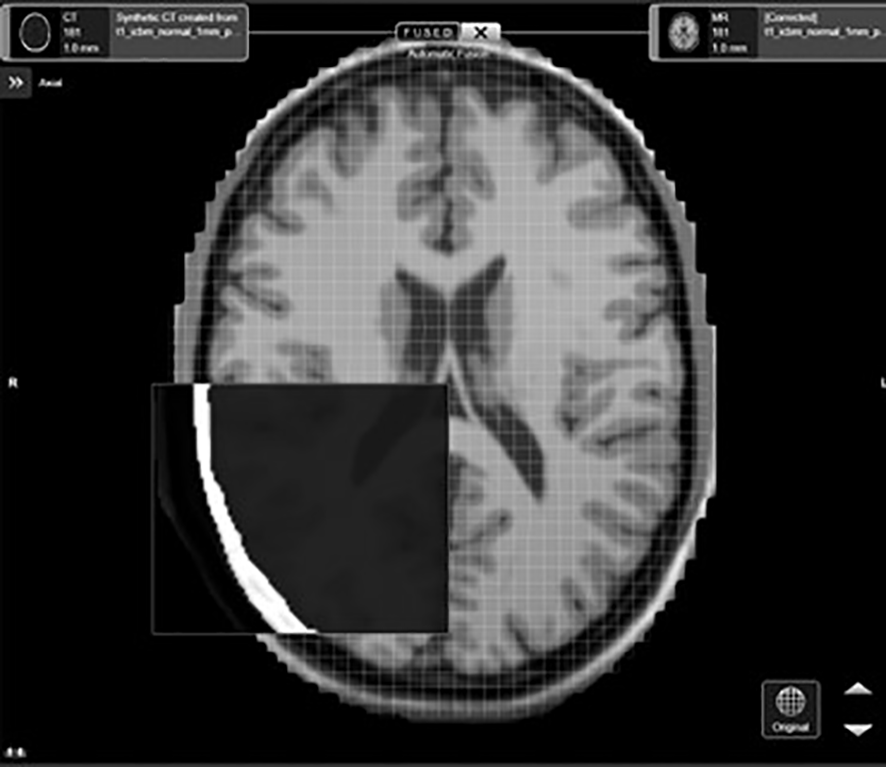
Display of the post-processed results of an MRI distorted dataset based on the synthetic CT using the cranial distortion correction software.
